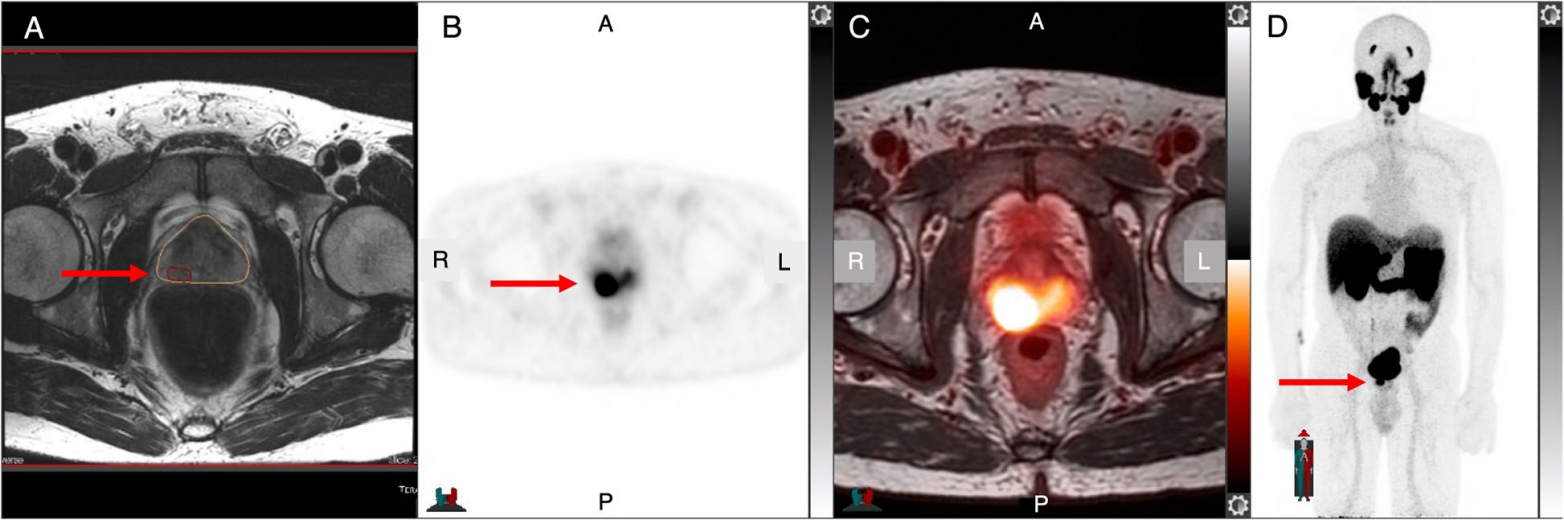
48-year-old man presents with PSA 11.30 ng/mL and PSA density 0.31 ng/mL^2^ for targeted prostate biopsy: mpMRI A shows a PI-RADS 4 lesion in the right lateral base whereas ^68^ Ga-PSMA11 axial PET B, axial fused PET/MRI C, and maximum intensity projection (MIP) D reveal a larger tumor volume. Subsequent PET-targeted biopsy resulted in a Gleason score 4 + 3 prostate cancer
